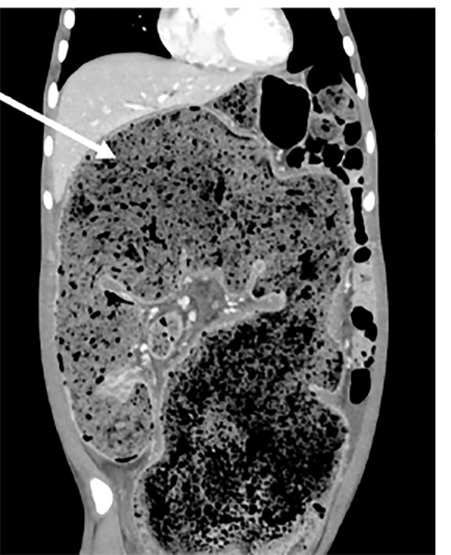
Sagital computed tomography with intravenous contrast demonstrating marked colonic distention with large stool burden.
